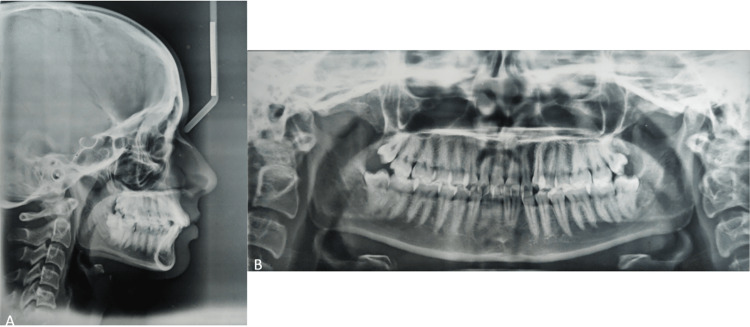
(A) Lateral cephalogram and (B) orthopantomogram depicting the absence of supernumerary or impacted teeth.
